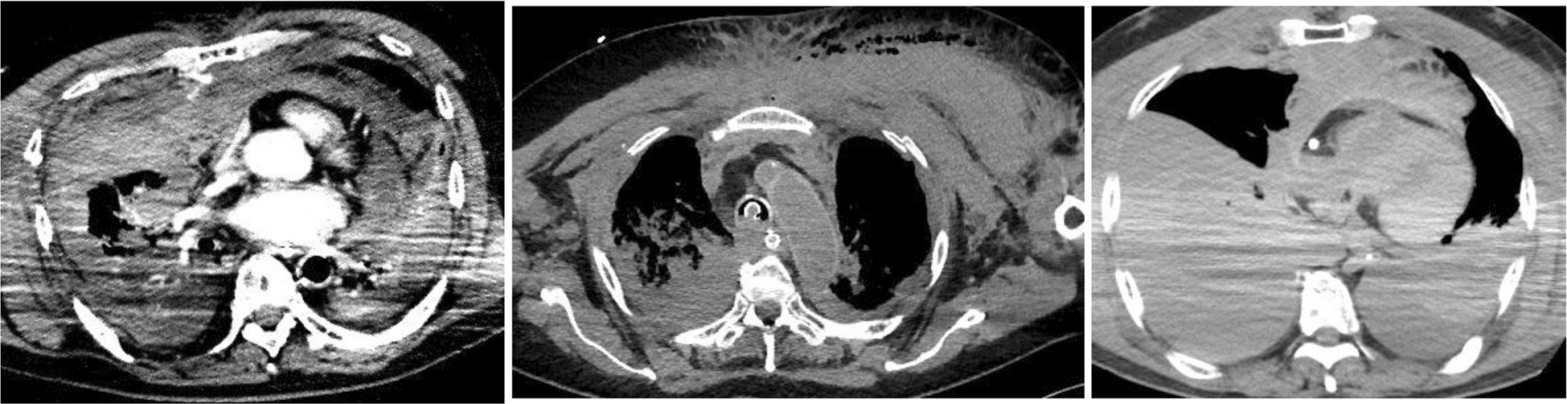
Computed tomography scans showing intra-thoracic, thoracic, and mediastinal bleeding, presumably caused by excessive chest compression, in the three patients in the present study who experienced fatal bleeding
